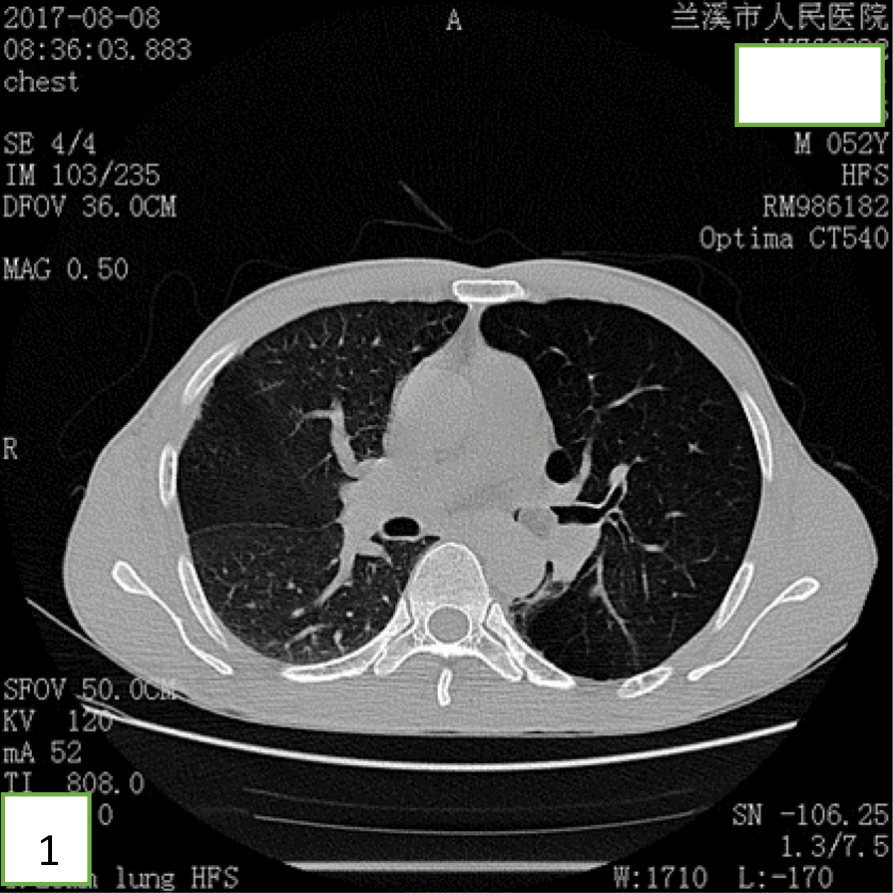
CT showing a low-density mass obstructing the left main bronchus, the CT value is -70HU
